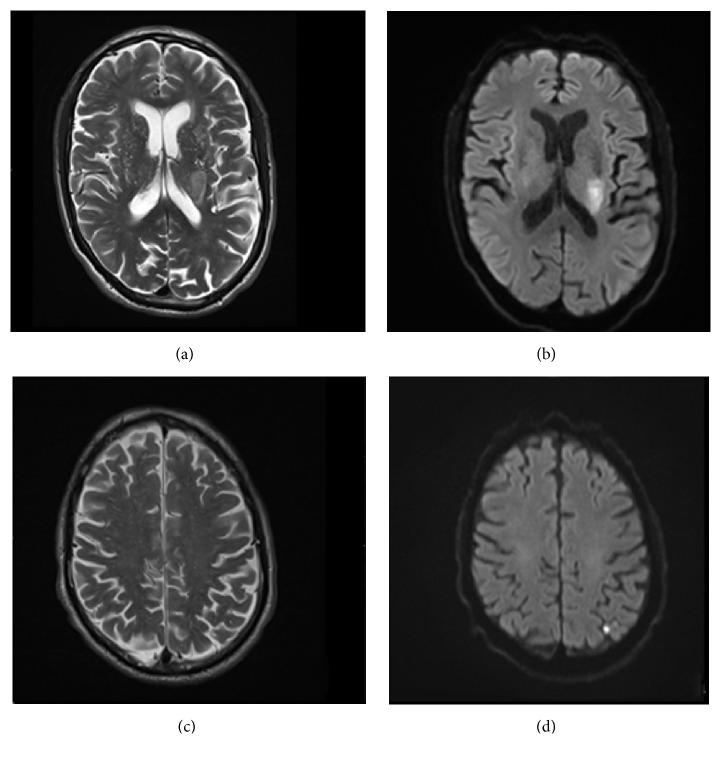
Brain MRI showing left corona radiata and parietal ischemic lesion on T2-weighted MRI (a, c) and diffusion-weighted imaging (DWI, (b), (d)).
